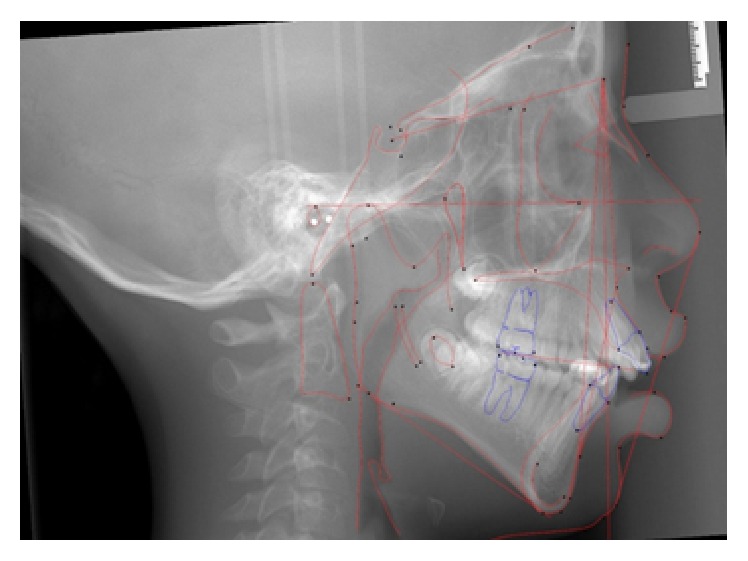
Initial cephalometric radiograph.
